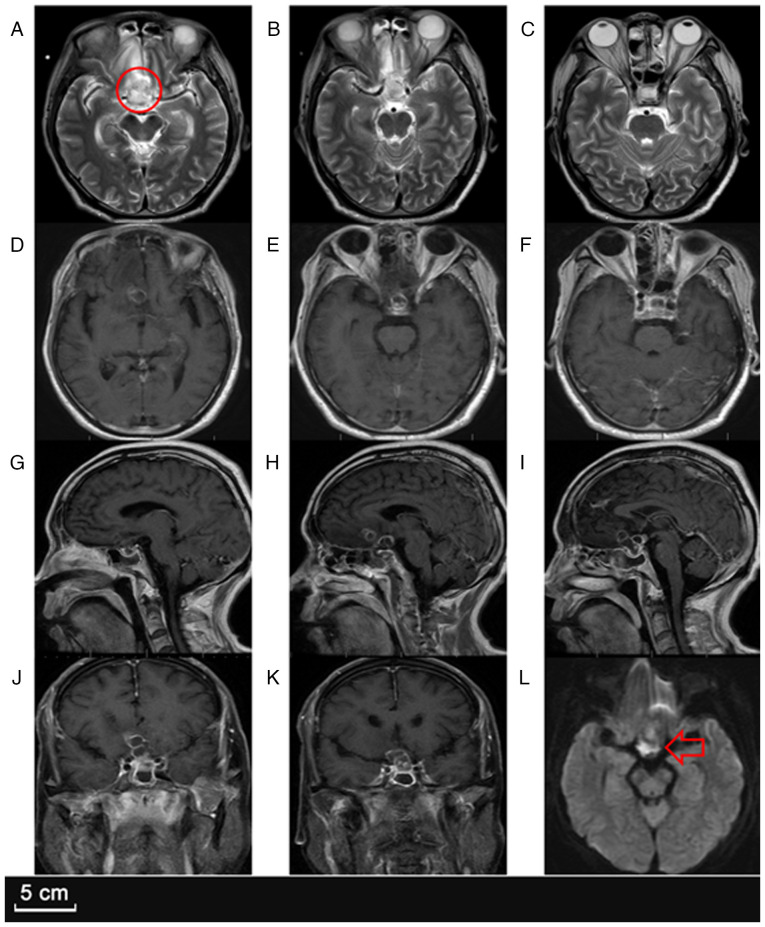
Preoperative MRI. (A) Axial T2-weighted MRI showing a larger area of mixed signal intensity in the sellar region (indicated by a circle) compared with the initial scan. (B and C) Axial T2-weighted MR images demonstrating increased perilesional edema and lesion expansion in both the sellar and suprasellar regions. (D-F) Axial T1-weighted contrast-enhanced MR images revealing annular enhancement in the sellar and frontal lobes, with an enlarged enhancement range compared to previous imaging. (G-I) Sagittal T1-weighted contrast-enhanced MR images showing progressive enhancement in the sellar and suprasellar regions as well as extension into the right frontal lobe. (J and K) Coronal T1-weighted contrast-enhanced MR images displaying more extensive ring-like enhancement in the sellar and right frontal areas. (L) On axial diffusion-weighted MR imaging, the saddle region (indicated by an arrow) exhibits a pronounced signal, suggesting evident limitations in dispersion.
